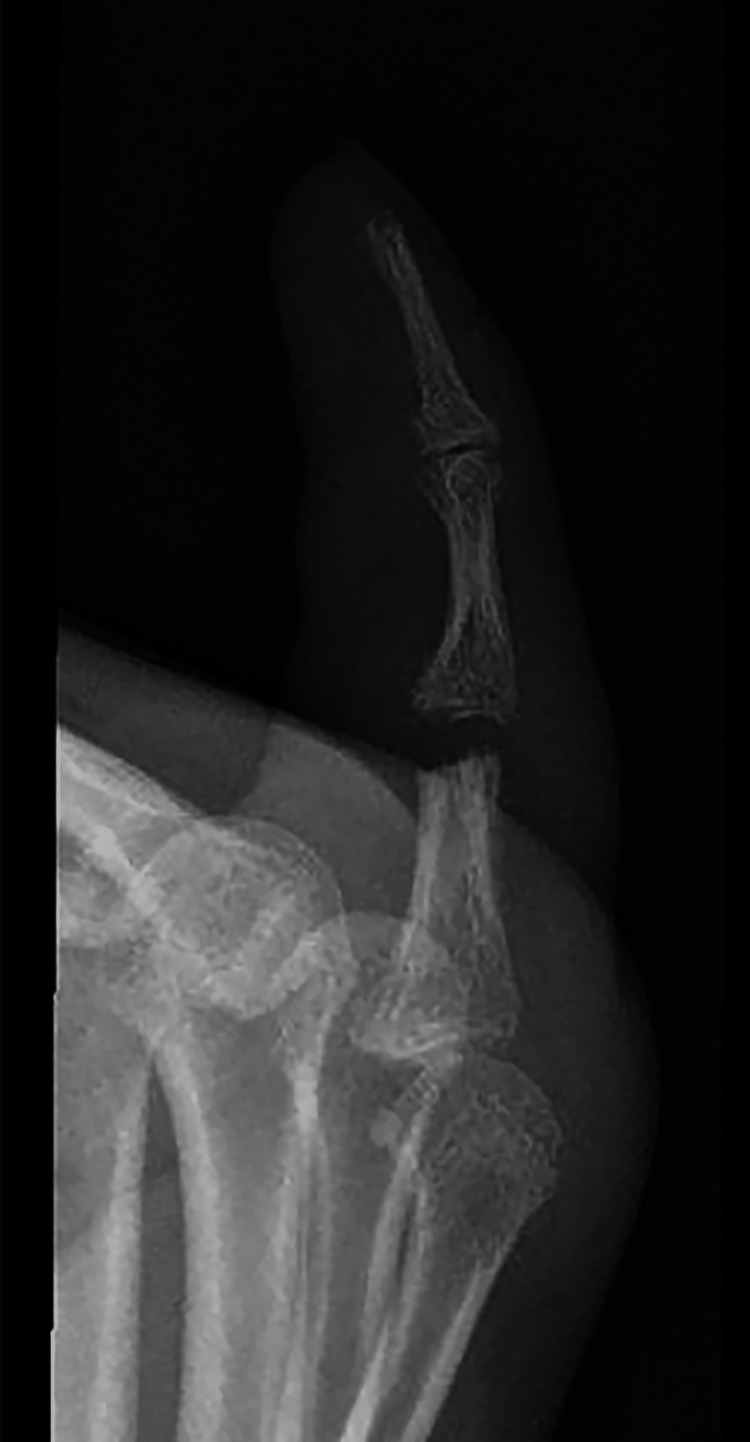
Postoperative radiographs, oblique view.
